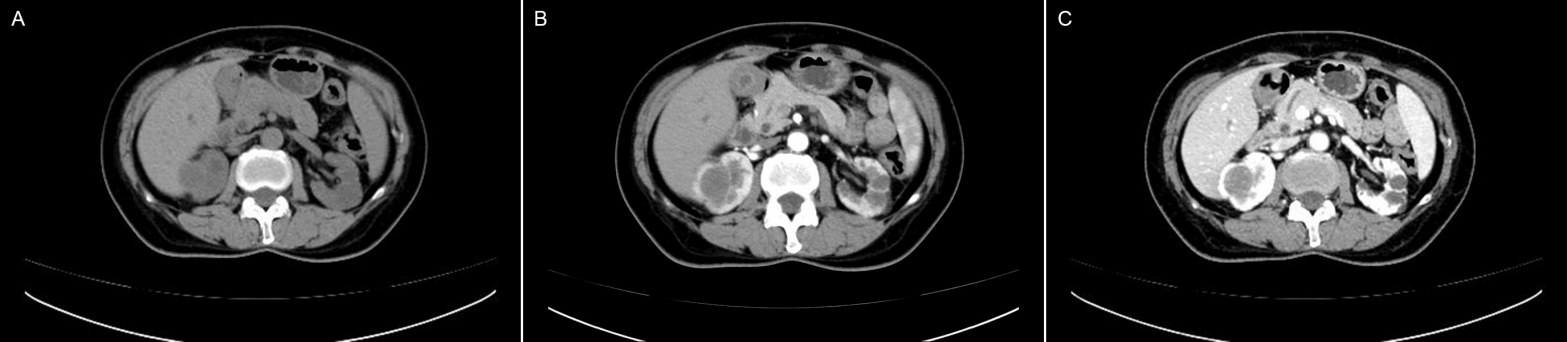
Computed tomography and contrast-enhanced computed tomography images of MTSCC: (A) CT plain scans showed a homogeneous slightly low density mass in the right kidney. Enhanced CT showed homogeneous mild delayed enhancement in arterial (B) and venous phases (C).
